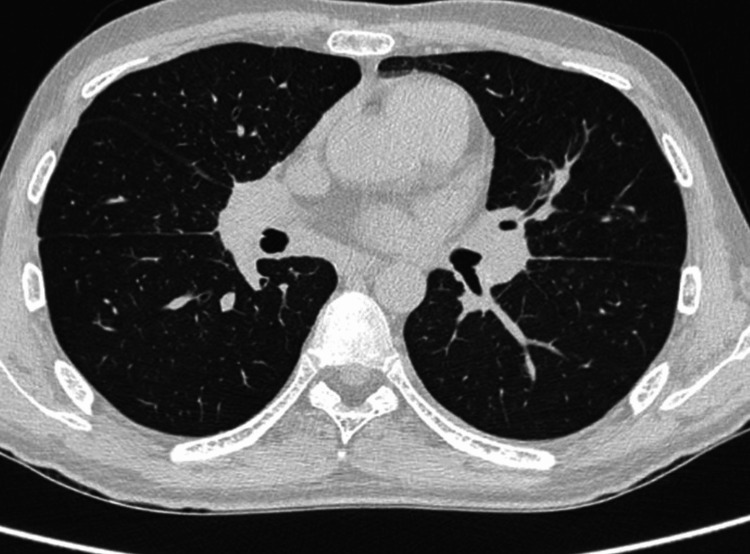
HRCT thorax post 4 months of steroid treatment. HRCT: high-resolution computed tomography
